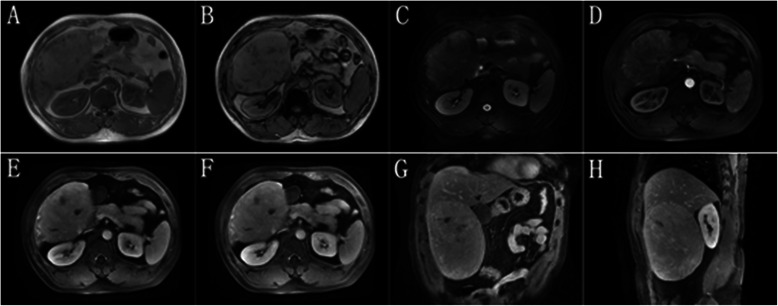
Magnetic resonance images show a large mass involving the right hepatic lobe. The mass with thickened tortuous vessels on the edge shows low signal intensity on T1-weighted dual-echo images (A and B), and slightly long signal intensity on T2-weighted images (C). D, in the arterial phase, heterogenous contrast enhancement is noted. E, in the portal venous phase, the heterogenous contrast enhancement of the tumor persists. F, the enhancement extent of the tumor remains greater than the liver parenchyma in the delayed phase. Coral image (G) and sagittal image (H) show the tumor and non-tumorous liver simultaneously. The liver is non-cirrhotic.
